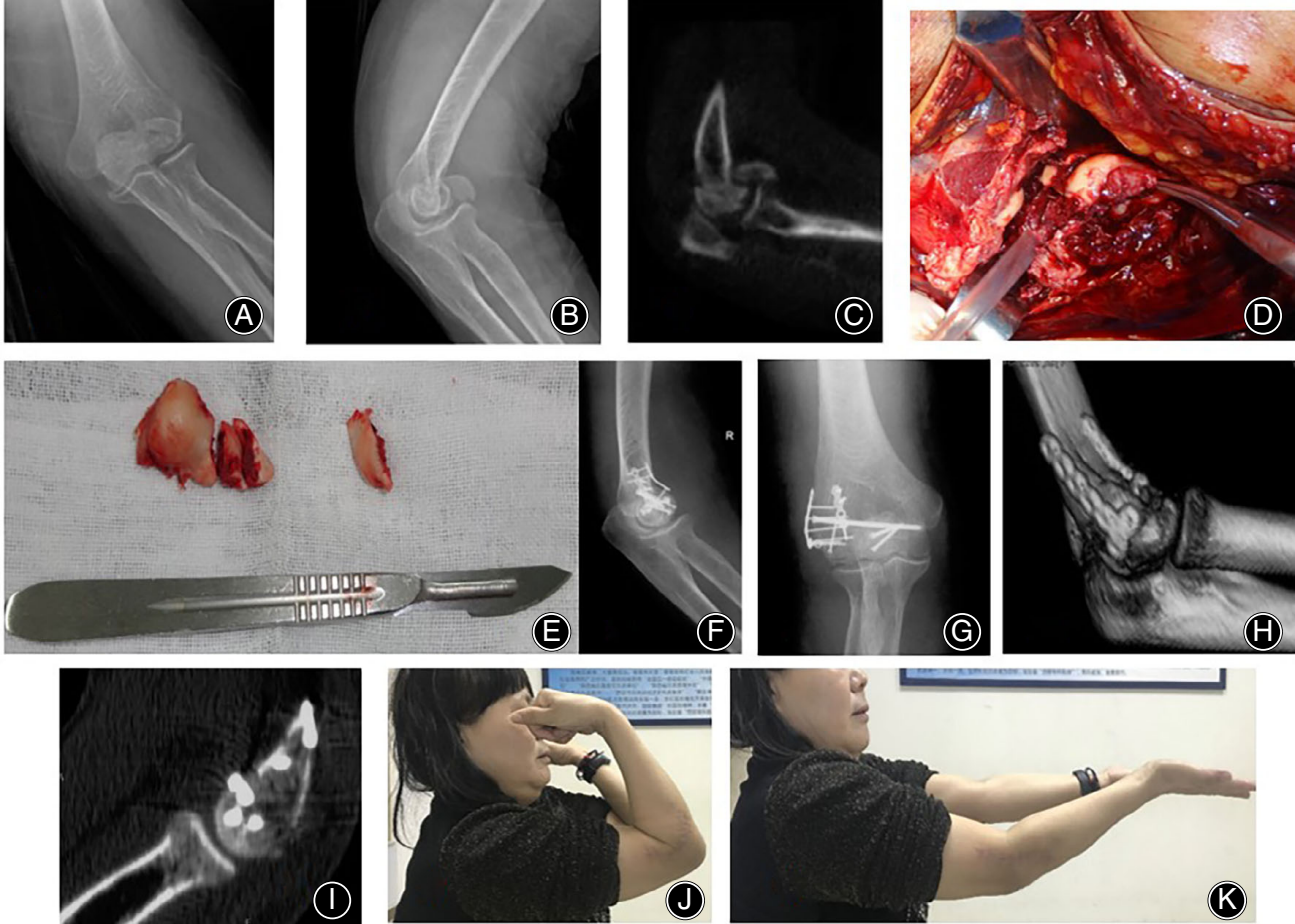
A 52‐year‐old female patient with capitellar fractures (Dubberley IIIB type) caused by fall from a height. Patient was treated with opening and micro‐locking plate fixation 10 days after injury. (A, B) Preoperative anteroposterior and lateral X‐ray examination showing frontal plane fracture of the distal end of the humerus; (C) Preoperative CT scans showing capitulum and trochlear fracture of the humerus; (D) Dissect and expose the fracture site; (E) The fragment is comminuted and dissociative sometimes; (F, G) Anteroposterior and lateral X‐ray is used to determine the fracture reduction and the placement of implants; (H, I) CT scans show satisfactory reduction and internal fixation; (J, K) functional appearance 1 year after operation showing satisfactory elbow function.
